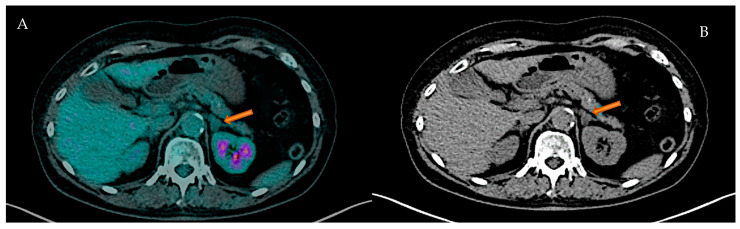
59-year-old female with history of NHL with a left adrenal-secreting mass indicated by arrows. (A): Axial [^18^F]FDG PET/CT fusion imaging shows a focal FDG uptake in left adrenal gland (SUV max: 2.13); (B): Axial CT low-dose imaging shows a low-density (UH −9.13) nodular lesion (maximum transverse diameter: 11 mm) in the left adrenal gland.
